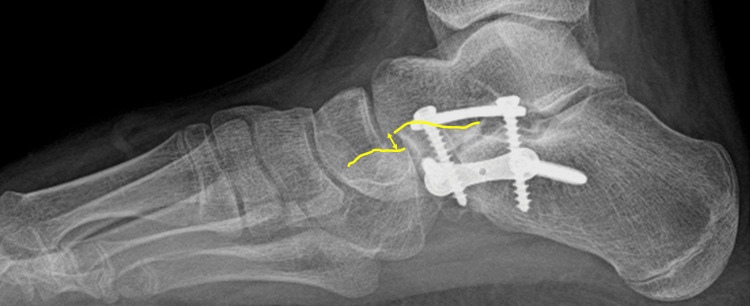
Measurement of CC joint subluxation on the lateral weight-bearing radiograph The CC joint subluxation is defined by the discontinuity between the upper dorsal calcaneal and the cuboid aspects and is measured by the distance between both of them (double arrow). CC: Calcaneocuboid
